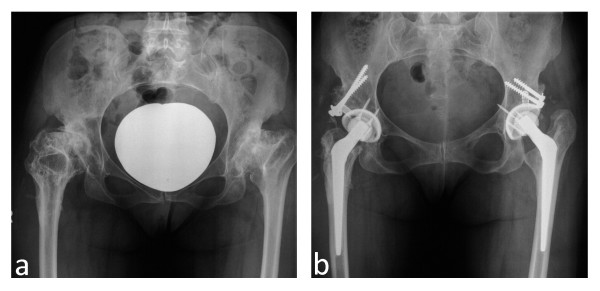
a-b: Anteroposterior pelvic radiograph of a 43 year old female with bilateral hip dysplasia and coxarthrosis. (a). Pelvic radiograph made five years after right and 6 years after left THA. The sockets are stable, and the bone grafts have healed (b).
